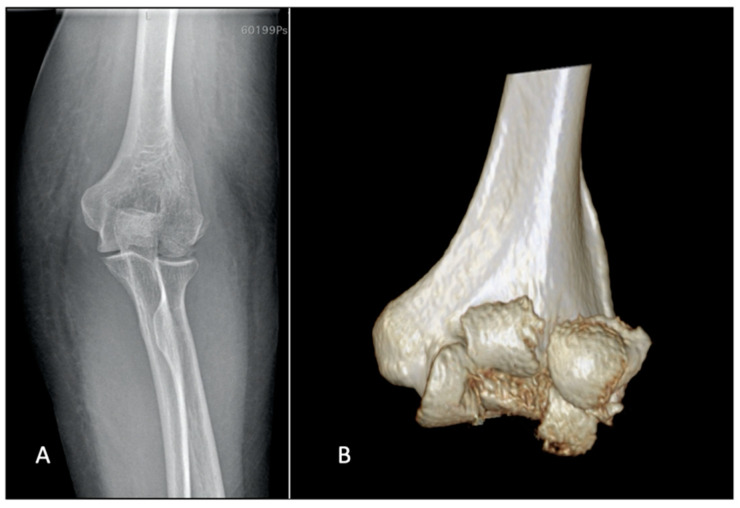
(A) The anteroposterior view barely shows involvement of the joint surface. (B) The CT scan portrays fracture of both the capitellum and the trochlea.
